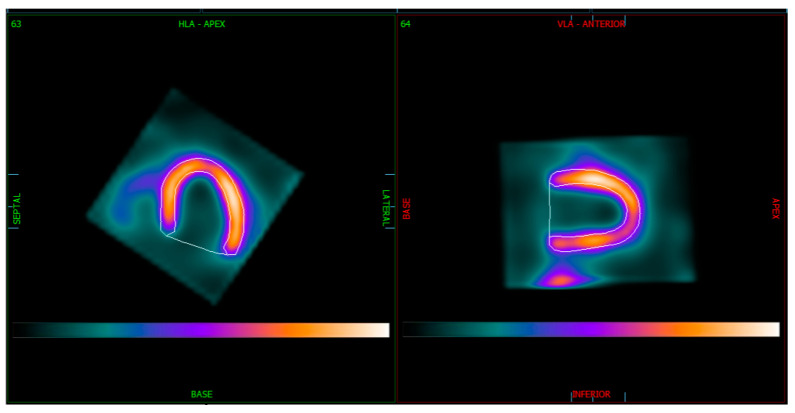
Veriton-CT SPECT images of LV myocardium in horizontal and vertical long axes with delineated contours.
